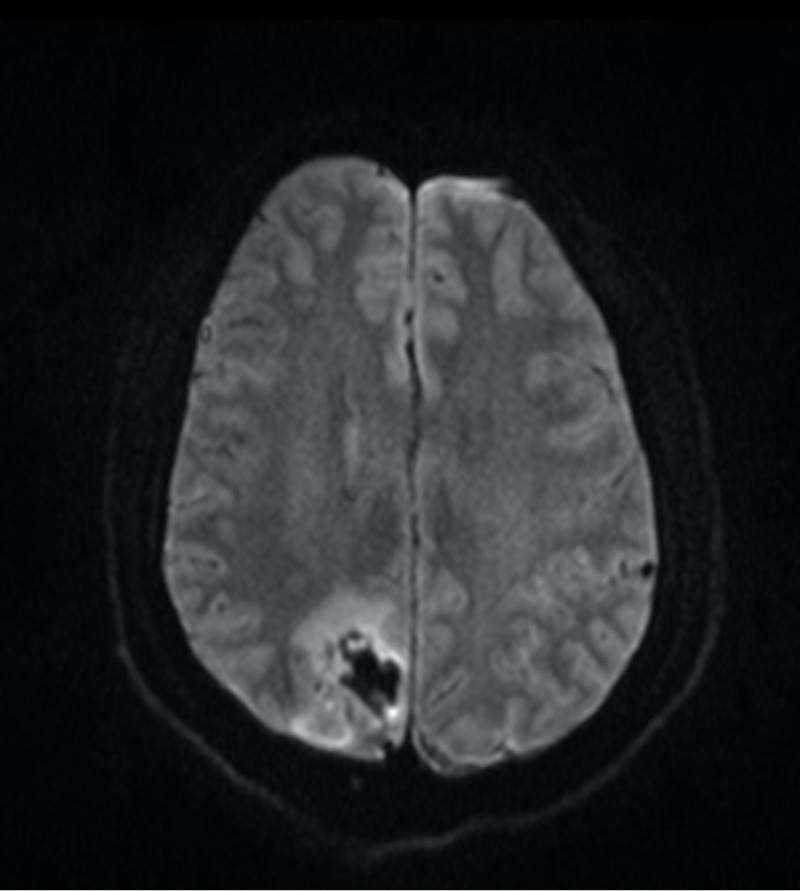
MRI brain, axial view. DWI with small areas of cortical restricted diffusion in the adjacent right paramedian posterior lobe.
